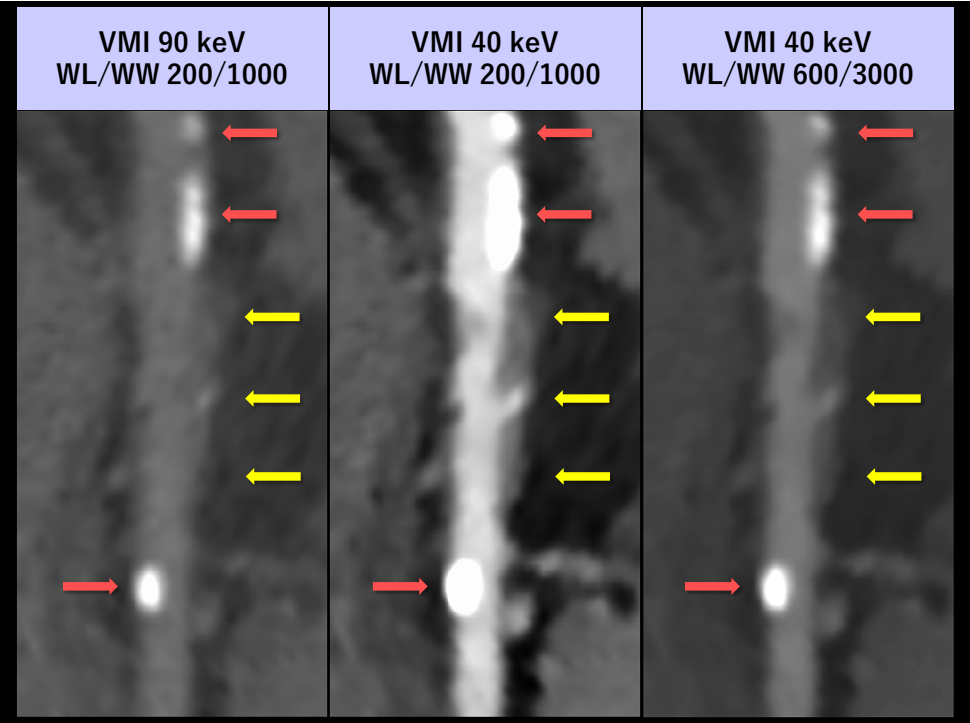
Stretched curved MPR of the right coronary artery in an 86-year-old man who underwent coronary CTA using dual-layer detector scanner with a low ICM dose (18 mL, 370 mgI/mL). The images were reconstructed with VMI at 90 and 40 keV. At the same window setting (WL/WW: 200/1000 HU), blooming artifacts from calcified plaques lead to an overestimation of luminal stenosis in the 40 keV VMI compared to the 90 keV VMI (red). However, mixed plaques with positive remodeling (yellow) are better visualized in the 40 keV VMI due to decreased attenuation of pericoronary fat and increased luminal attenuation. By optimizing the window setting for 40 keV, the degree of visual blooming artifacts from calcified plaques remains consistent with those at 90 keV, while luminal brightness, sharpness, and depiction of mixed plaques with positive remodeling are superior in the 40 keV images compared to the 90 keV images
